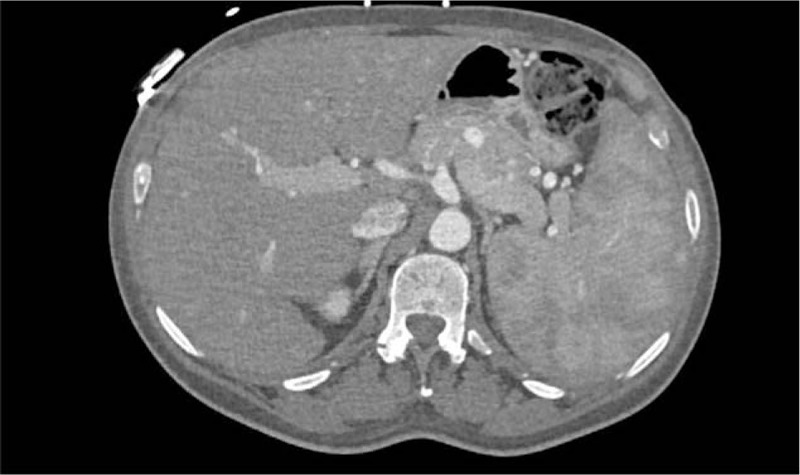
Severe splenomegaly at CT abdomen scan.
